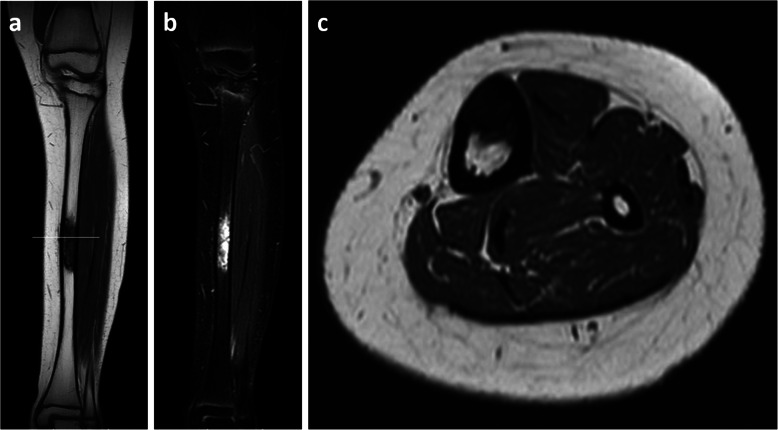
The mass was a heterogeneously hypointense and isointense on a T1-weighted magnetic resonance image and b heterogeneously hyperintense on short TI inversion recovery magnetic resonance image. No pathological fracture was confirmed
